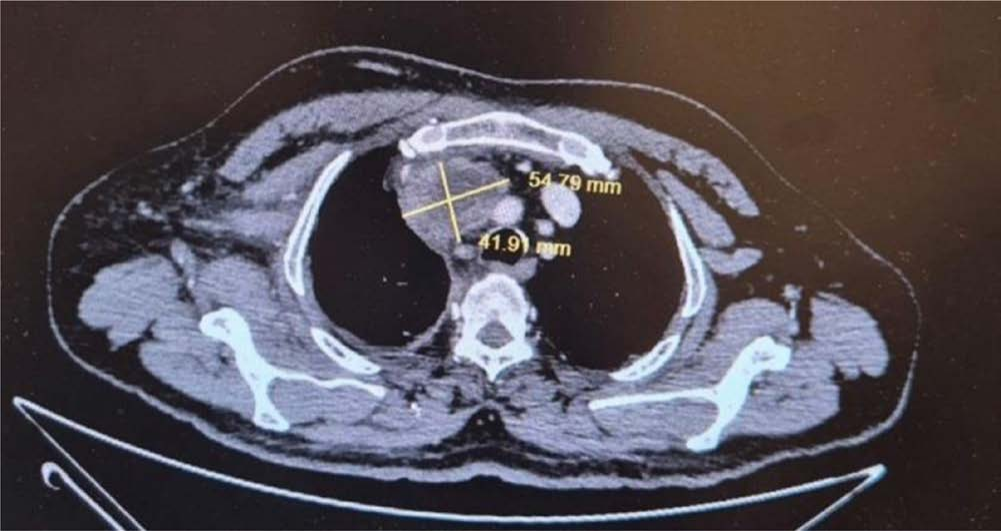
The patient’s contrast-enhanced CT image. Contrast-enhanced thoracic computed tomography (CT) demonstrating a well-defined soft tissue mass measuring approximately 55 × 40 mm in the right hilar region, compressing the adjacent subclavian vein and upper mediastinal vascular structures.
